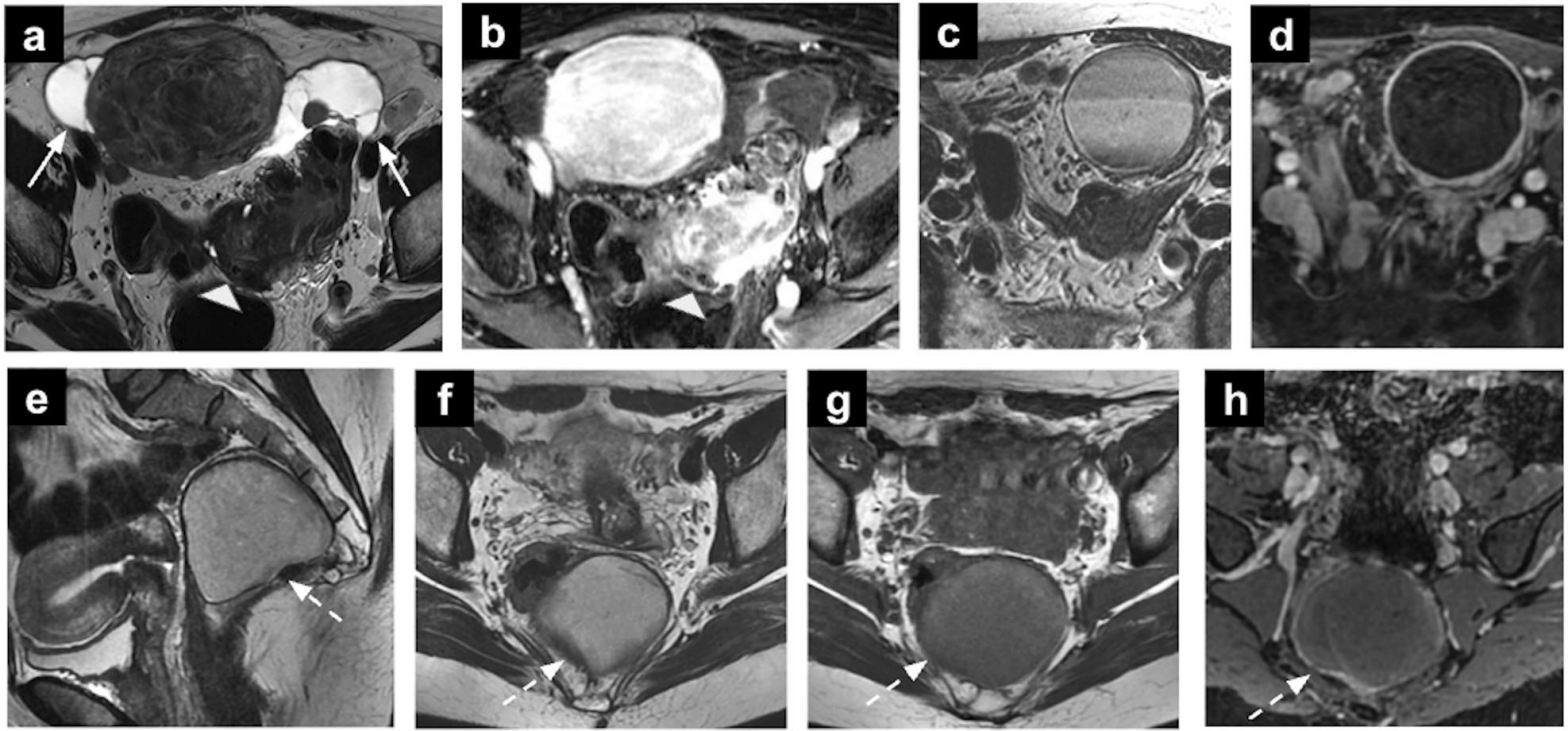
Peritoneal inclusion cysts and diverticulitis (a, b). Axial T2-WI (a) and post-contrast T1-WI (b) showing inclusion cysts (arrows) in a 63-year-old patient undergoing MRI for para-ovarian cysts characterisation. They appear as thin-walled and fluid-filled structures without contrast enhancement, conform to the surrounding uterus and ovaries, due to their reactive nature to peritoneal adhesions. Differential considerations include mesenteric cysts (c, d), which, in contrast, are typically isolated and do not conform to adjacent structures. In this case of peritoneal inclusion cysts, there is also evidence of diffuse sigmoid wall thickening (arrowhead in a) with avid enhancement (arrowhead in b), in keeping with concomitant diverticulitis. Tailgut cyst (e–g). Sagittal and axial T2-WI (e, f), axial T1-WI (g) and post-contrast T1-WI (h) showing a tailgut cyst (dotted arrow). It appears as a retrorectal thin-walled unilocular cystic lesion with high-SI and intermediate-to-low-SI content on T2-WI (e, f) and T1-WI (g), respectively. No enhancing component is noted. SI, signal intensity; WI, weighted images
